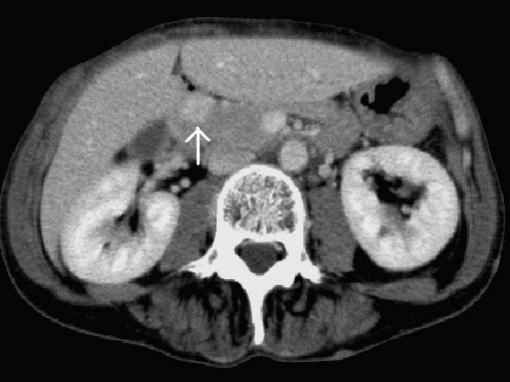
An enhanced computed tomography scan revealing a hypervascular tumor in the head of the patient's pancreas (arrow).
